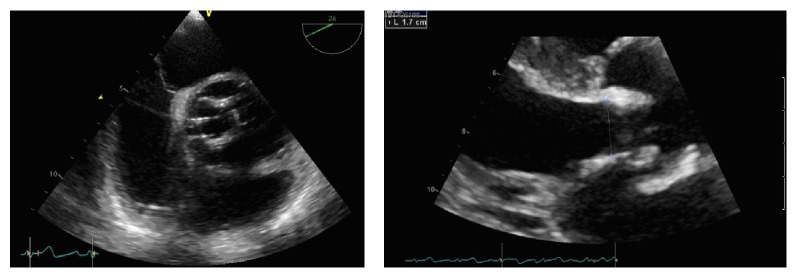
Transesophageal echocardiography images showing thickness of the biological valvar prosthetic leaflets.
